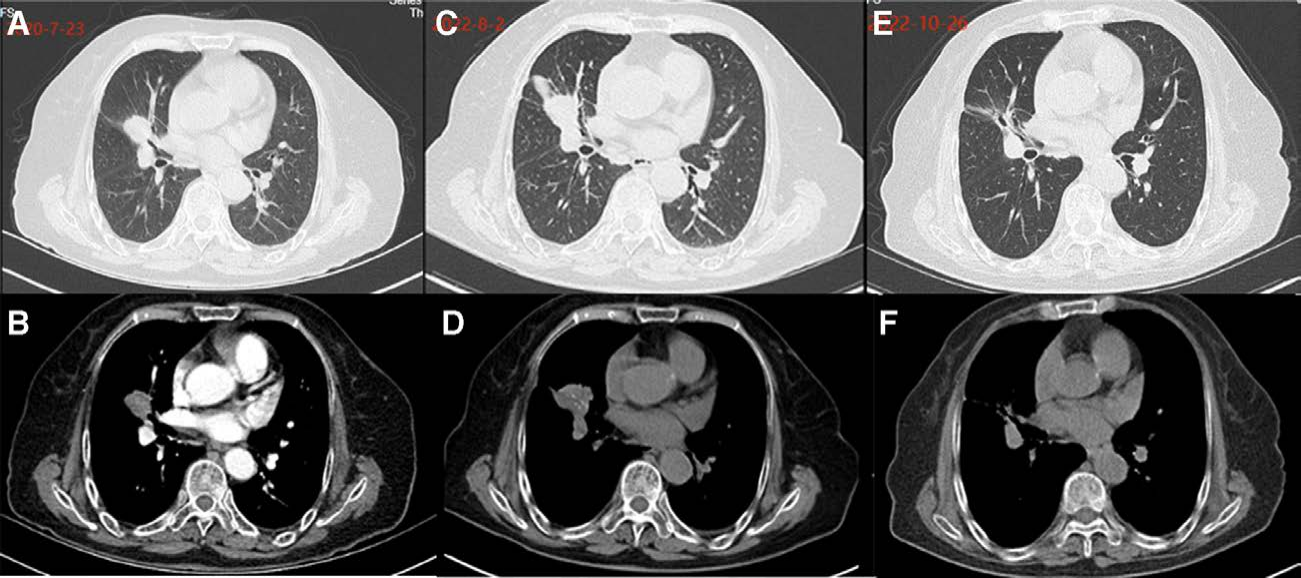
Comparison of CT images of both lungs before and after crizotinib treatment. (A and B) Neoplasm occupying the lateral segment of the right lung middle lobe. (C and D) Adenocarcinoma was diagnosed without treatment, and the disease progressed 2 years later. (E and F) After 2 months of treatment with crizotinib, the tumor was significantly smaller than before. CT = computed tomography.
